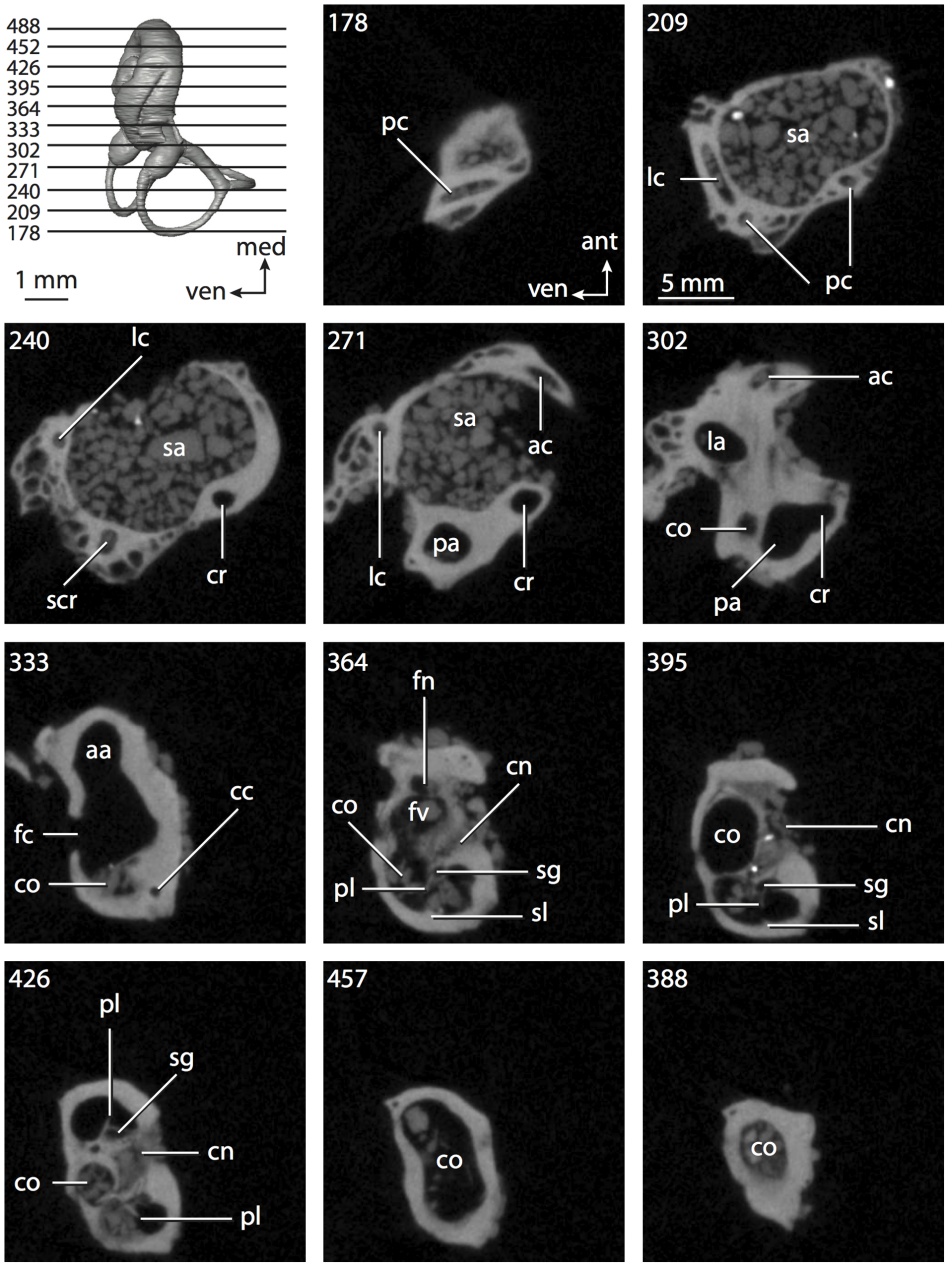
CT slices through ear region of*Kulbeckia kulbecke.* Numbers refer to specific CT slices. Additional information provided by Ekdale and Rowe [83]. Abbreviations listed at the end of the Materials and Methods section.
